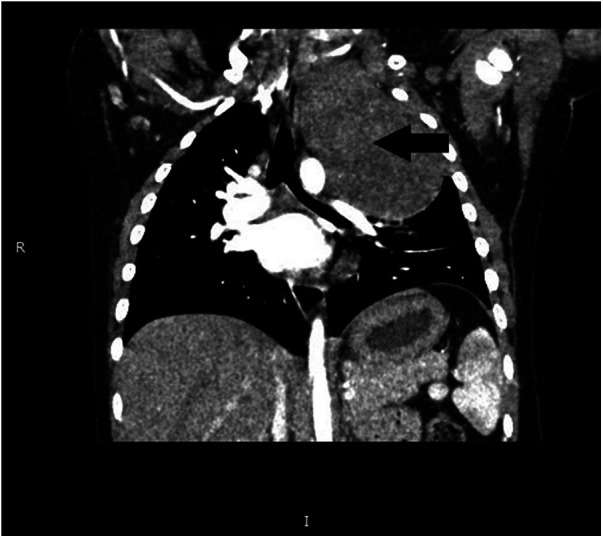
Chest CT results of the patient. Arrow: mediastinal tumor.
